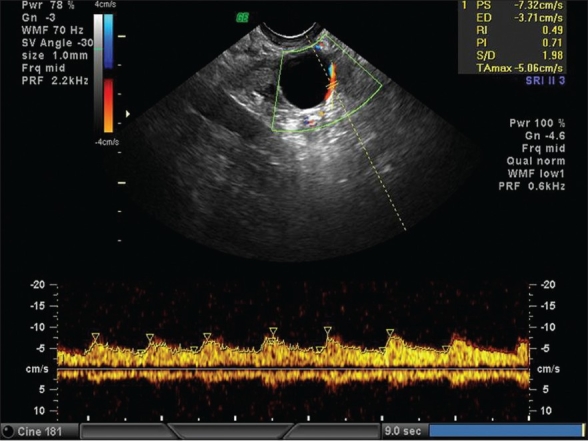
Preovulatory follicle with pulse doppler showing low resistance flow
